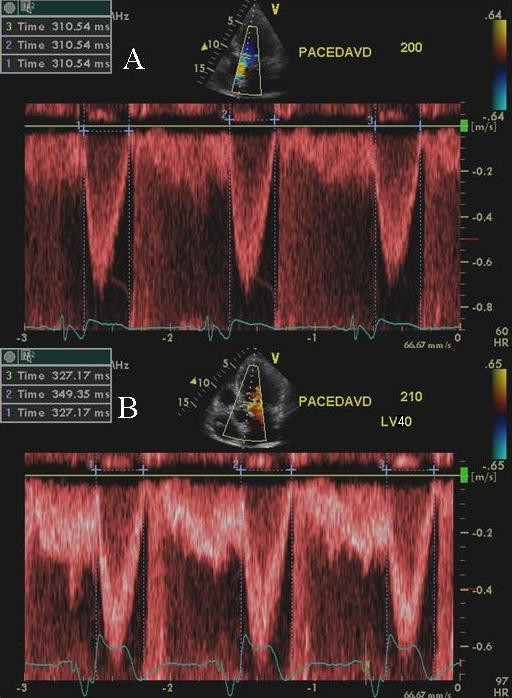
PW Doppler showing left ventricular outflow tract velocity. Improvement in left ventricular ejection duration is shown in B during LV pre-excitation of 40 ms compared to baseline VV delay of 0 ms in A.
